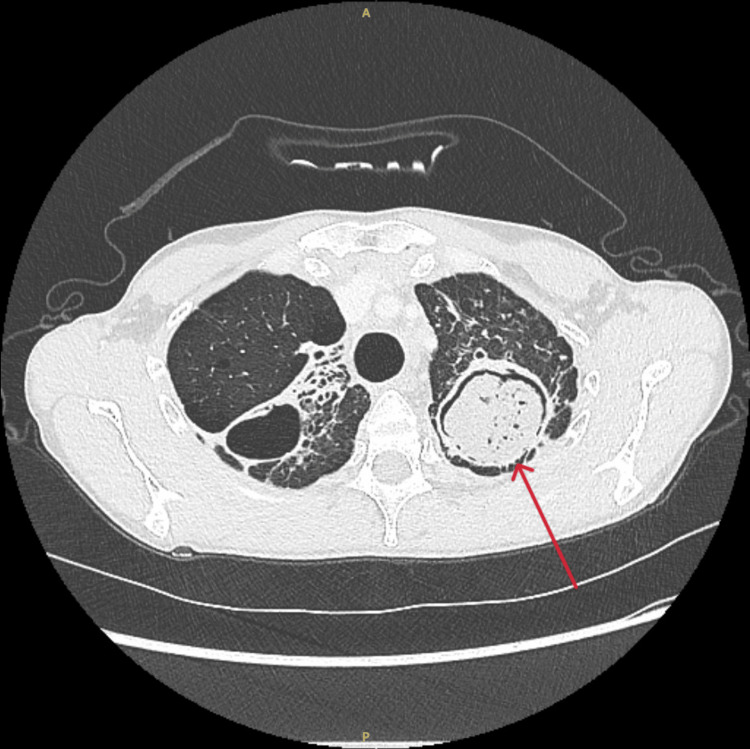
Computed tomography of the chest shows an aspergilloma in the left upper lobe.
